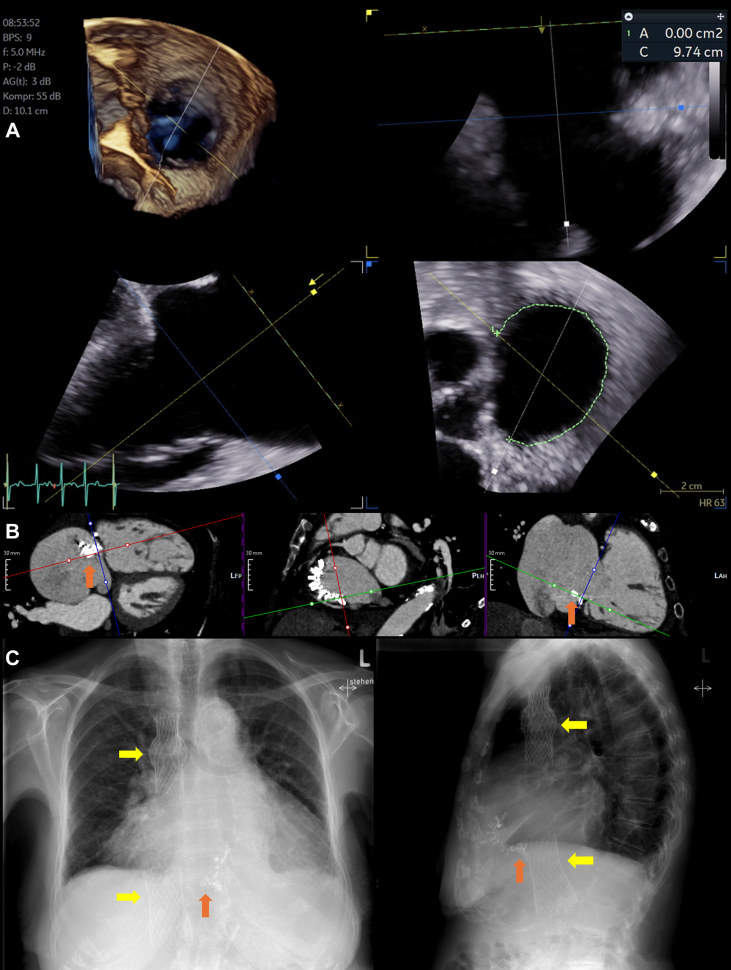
Pre-, Peri- and Postinterventional Imaging for Patient 2 (A) Three-dimensional transesophageal echocardiography demonstrates annuloplasty coverage measurement of the tricuspid annulus, starting anteriorly, as close to the aorta as possible, right to the opening of the coronary sinus. (B) Cardiac computed tomography angiography multiplanar reconstruction demonstrates Cardioband dislocation of the annuloplasty device (orange arrows). (C) Postprocedural chest radiography (anteroposterior and lateral views) show placement of the superior vena cava and inferior vena cava valves (yellow arrows) as well as the dislocated annuloplasty device (orange arrows).
